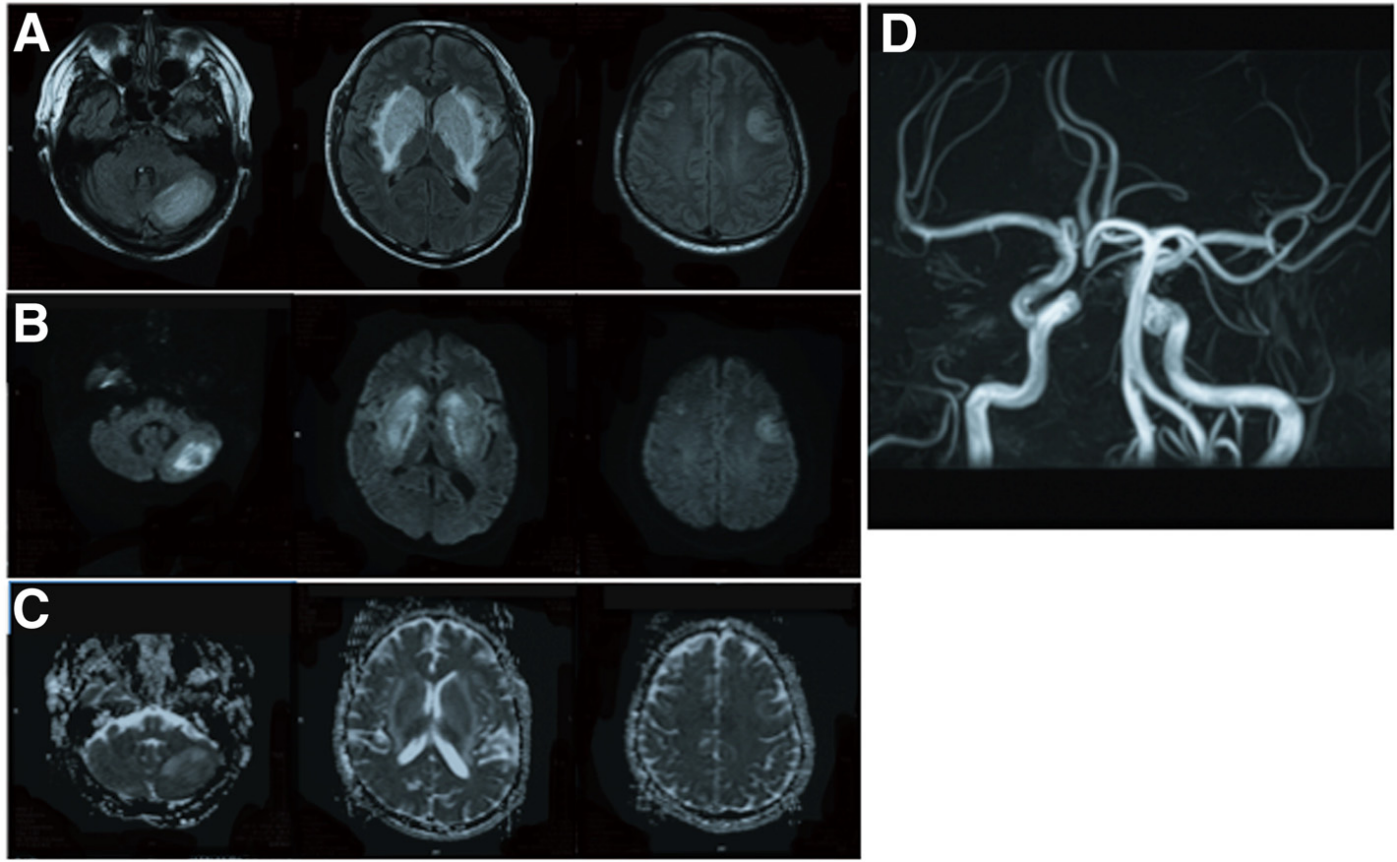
MR images on admission. Representative images of brain MRI including fluid attenuated inversion recovery (FLAIR) (A), diffusion-weighted image (DWI) (B), apparent diffusion coefficient (ADC) map (C), and MR angiography (D) on admission, showing high-signal-intensity lesions in the bilateral basal ganglia, left cerebellar hemisphere, and bilateral frontal cortices.
